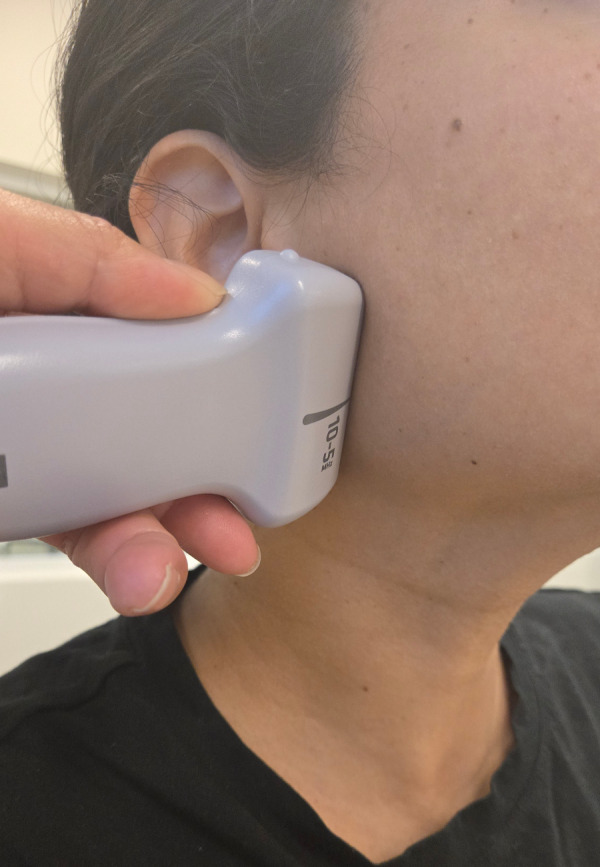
Longitudinal scanning plane of the parotid gland using point of care ultrasound (POCUS).
